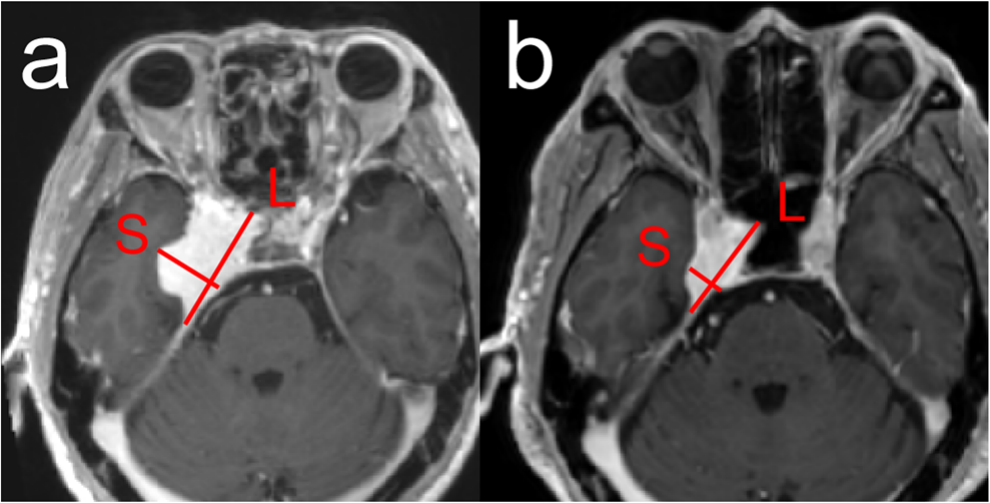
Radiographic change in a representative case. a Contrast-enhanced T1-weighted magnetic resonance imaging (MRI) image of a cavernous sinus meningioma before conventionally fractionated stereotactic radiotherapy (CFSRT). b Contrast-enhanced T1-weighted MRI 35 months after CFSRT. L, long-axis diameter of the tumor; S, short-axis diameter of the tumor
